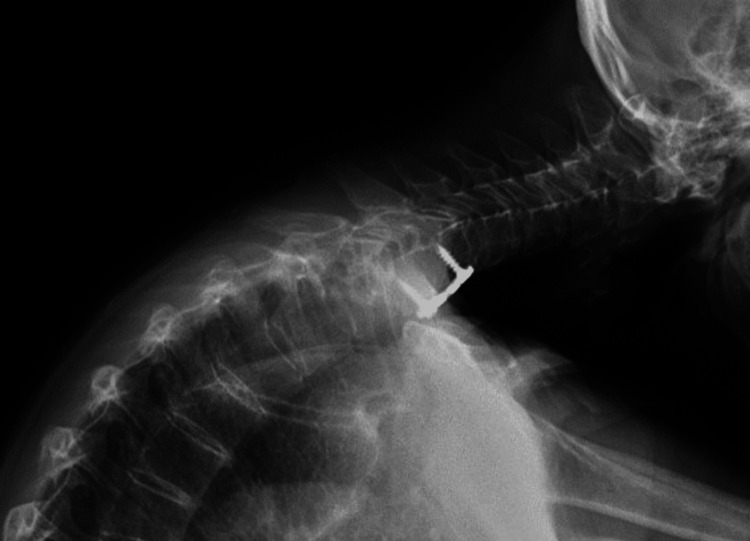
X-ray of patient in relaxed lateral position six months post DHS onset.
